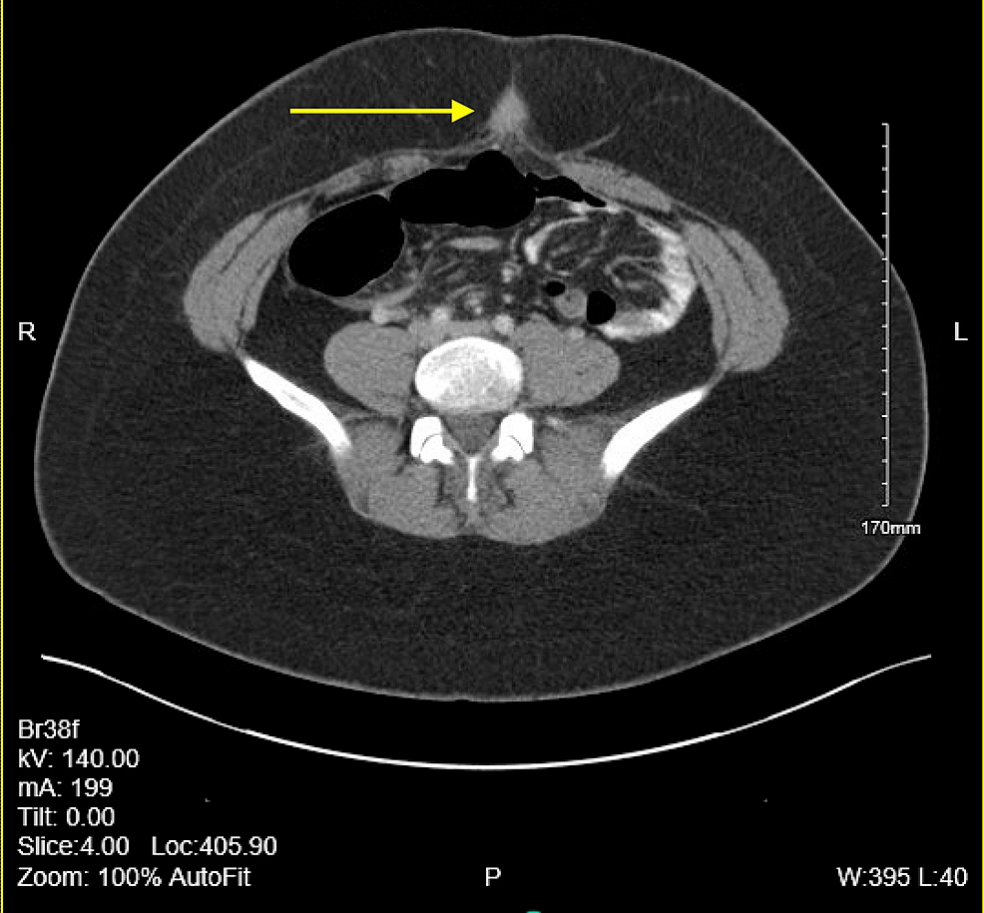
Computerized tomography (CT) scan 1
